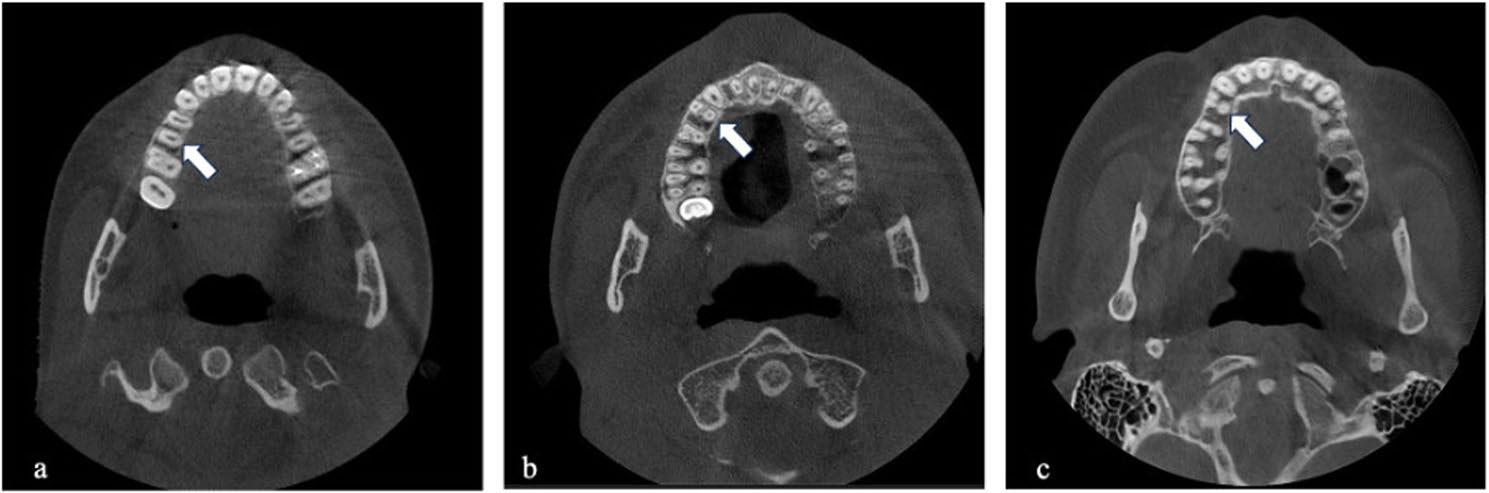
Axial CBCT images of the number of roots only in maxillary premolar teeth. (a) One root, (b) two roots, (c) three roots
